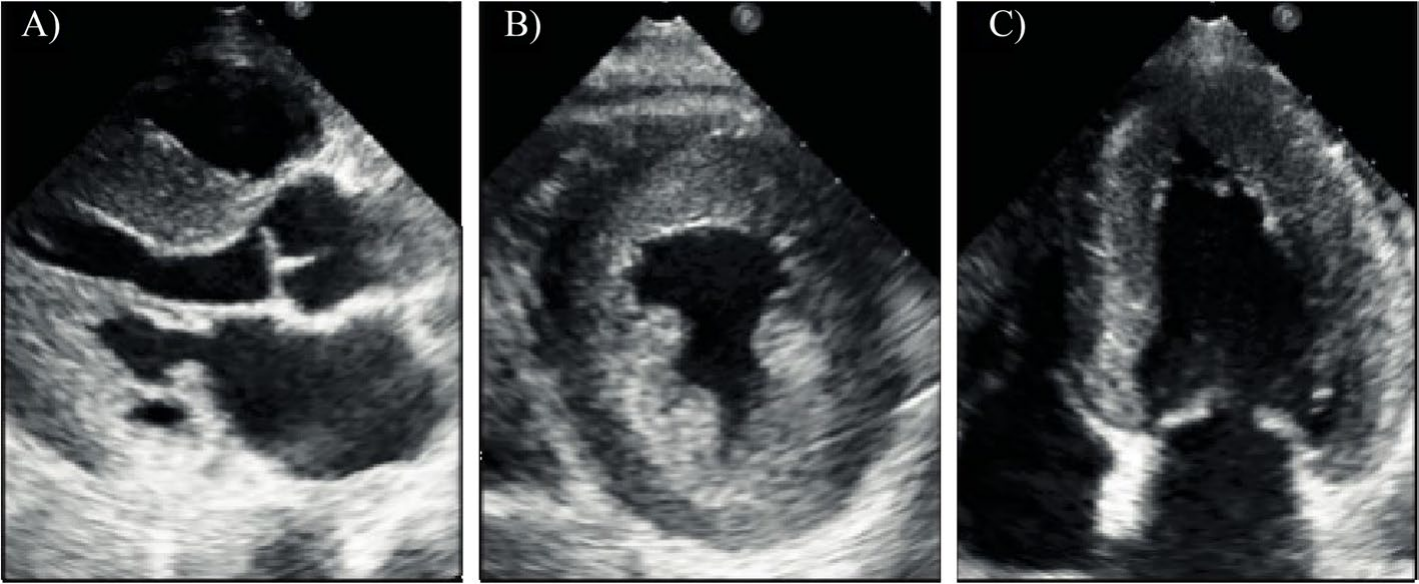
Echocardiographic views. Parasternal long-axis (A), parasternal short axis (B) and apical four chambers (C, frames taken during diastole). The exam revealed the presence of non-dilated left ventricle affected by marked concentric hypertrophy with ground glass appearance
